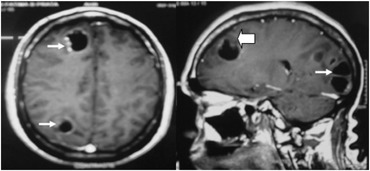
Magnetic resonance imaging (MRI) showing rounded cysts characterized by low signal on T1-weighted sequences and hyperintense on T2-weighted sequences (thin arrows). Some cysts have small nodules on their wall (large arrow). Note the contrast enhancement on the periphery of the cyst and more intensely in the region of mural nodules.
